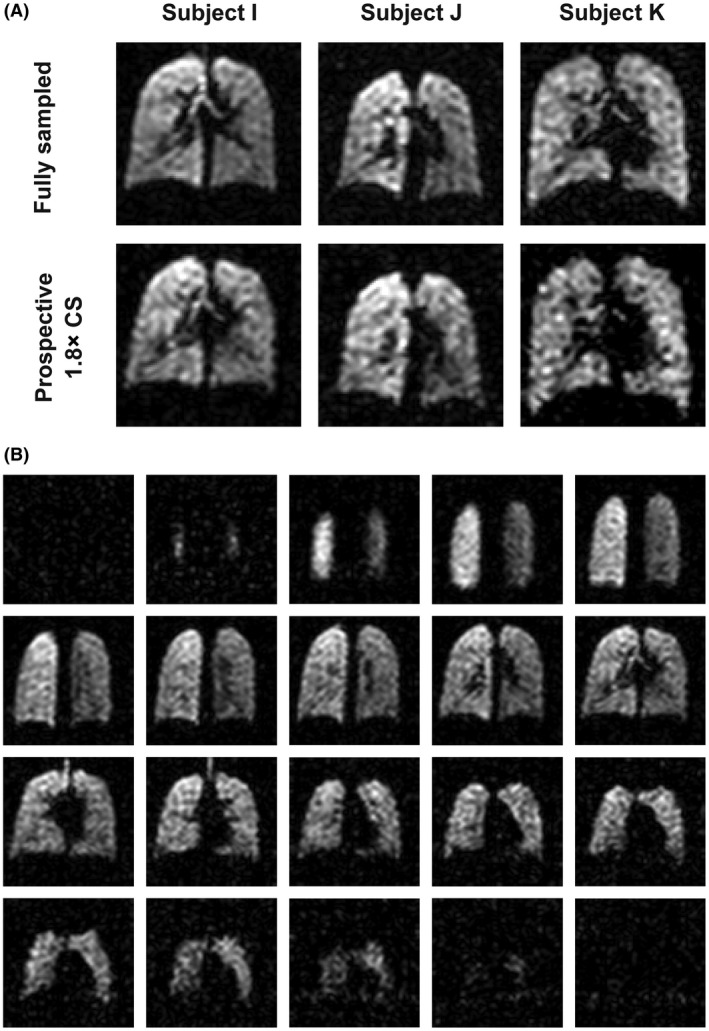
A, Coronal slices from 3D fully sampled and prospectively 1.8× accelerated scans (NSA = 4), acquired from three healthy volunteers in separate breath holds. Difference images are not shown because of lack of registration between images resulting from minor difference in lung inflation levels between breath holds). B, A 3D ^19^F‐MRI scan acquired with 1.8× prospective acceleration (NSA = 4) in a scan of 10‐s duration, showing whole‐lung coverage at 1‐cm isotropic resolution; NSA, number of signal averages
